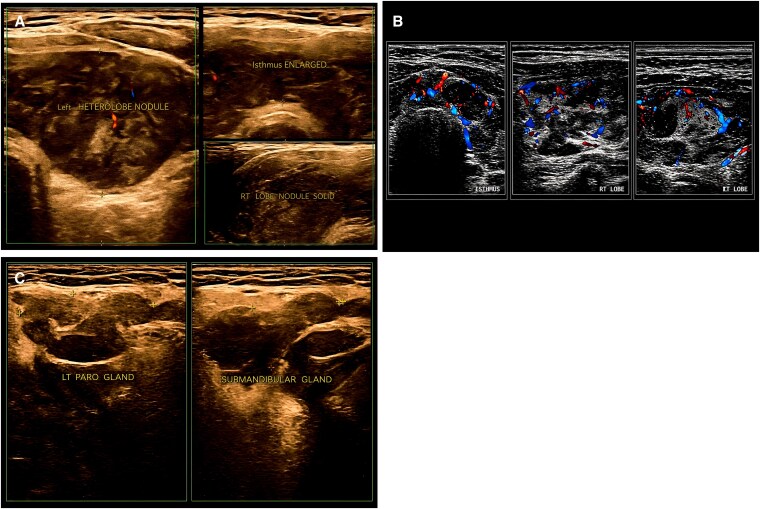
(A) USG of the thyroid gland showing diffuse enlargement and nodules. (B) Color Doppler of the thyroid gland showing increased vascularity. (C) USG showing submandibular gland and parotid gland enlargement.
